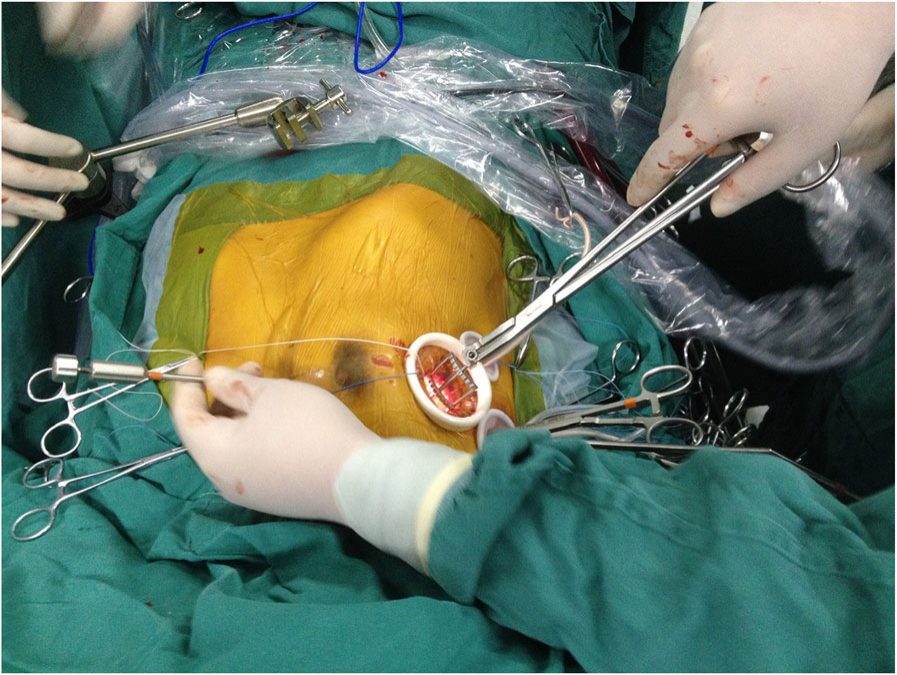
Left atrium exposure by means of a blade retractor. The blade retractor was inserted through port 2 to raise the left atrium with a shaft. The blade retractor was fixed on a 2-mm shaft that penetrated parasternally in the fourth intercostal space and was held on the operating table
